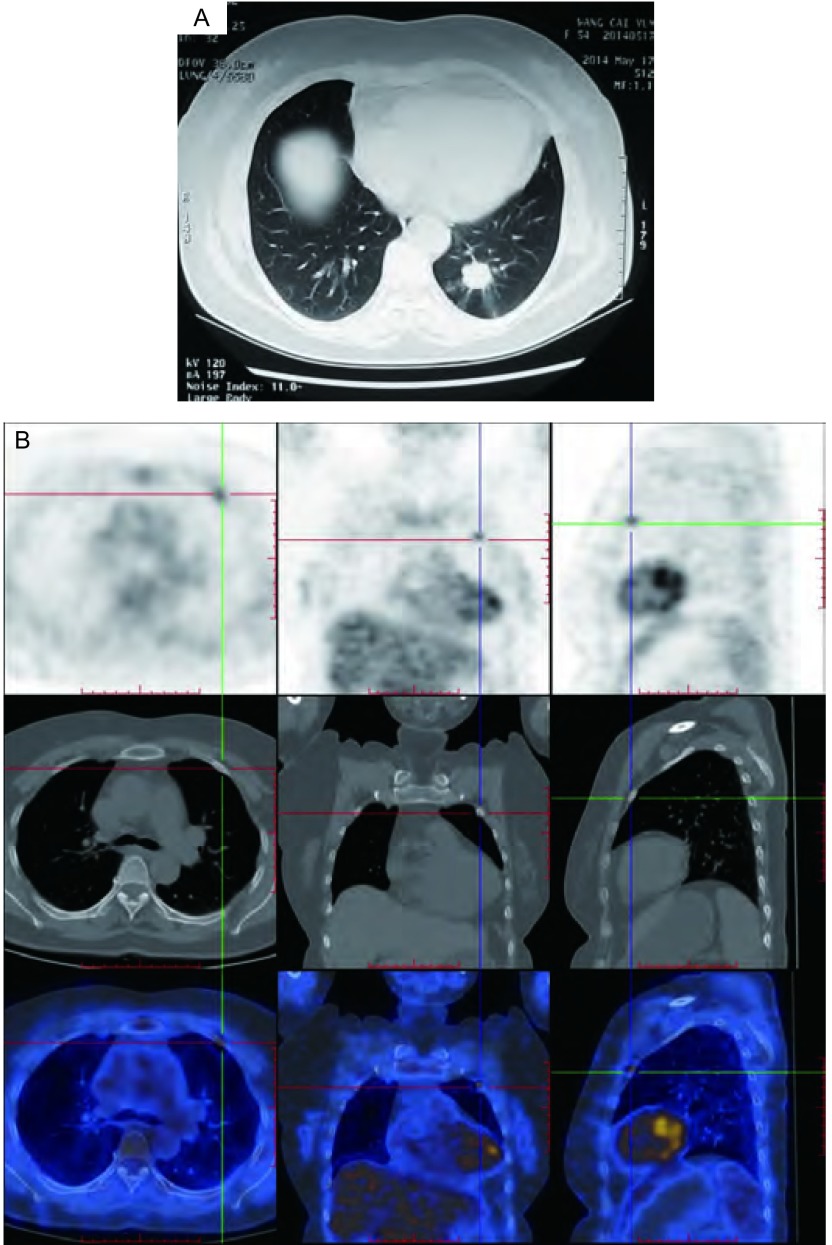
患者临床影像学特征。A：胸部CT显示左肺下叶肿瘤。B：PET-CT示左侧第二肋骨溶骨性病变伴代谢增高。 Clinical radiologic features of the patient. A: Chest CT scan image demonstrating the tumor located in left lower lobe. B: PET-CT scan demonstrating osteolytic lesion in the left 2^nd^ rib with heterogeneous radiotracer uptake. CT: computed tomography; PET-CT: positron emission tomography-computed tomography.
